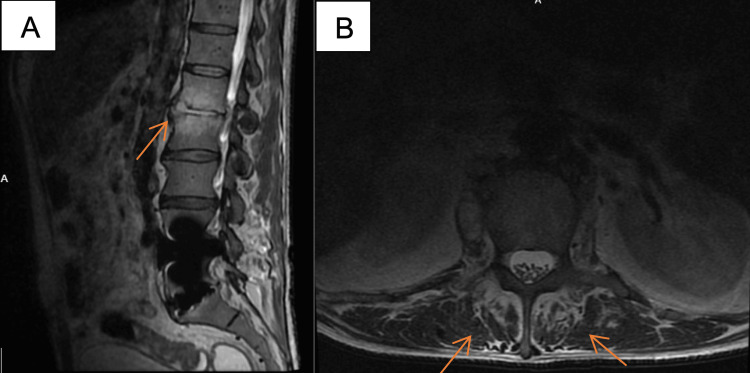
MRI lumbar spine T2 (A) L1-L2 osteomyelitis on sagittal view; (B) bilateral psoas abscesses on axial view.
